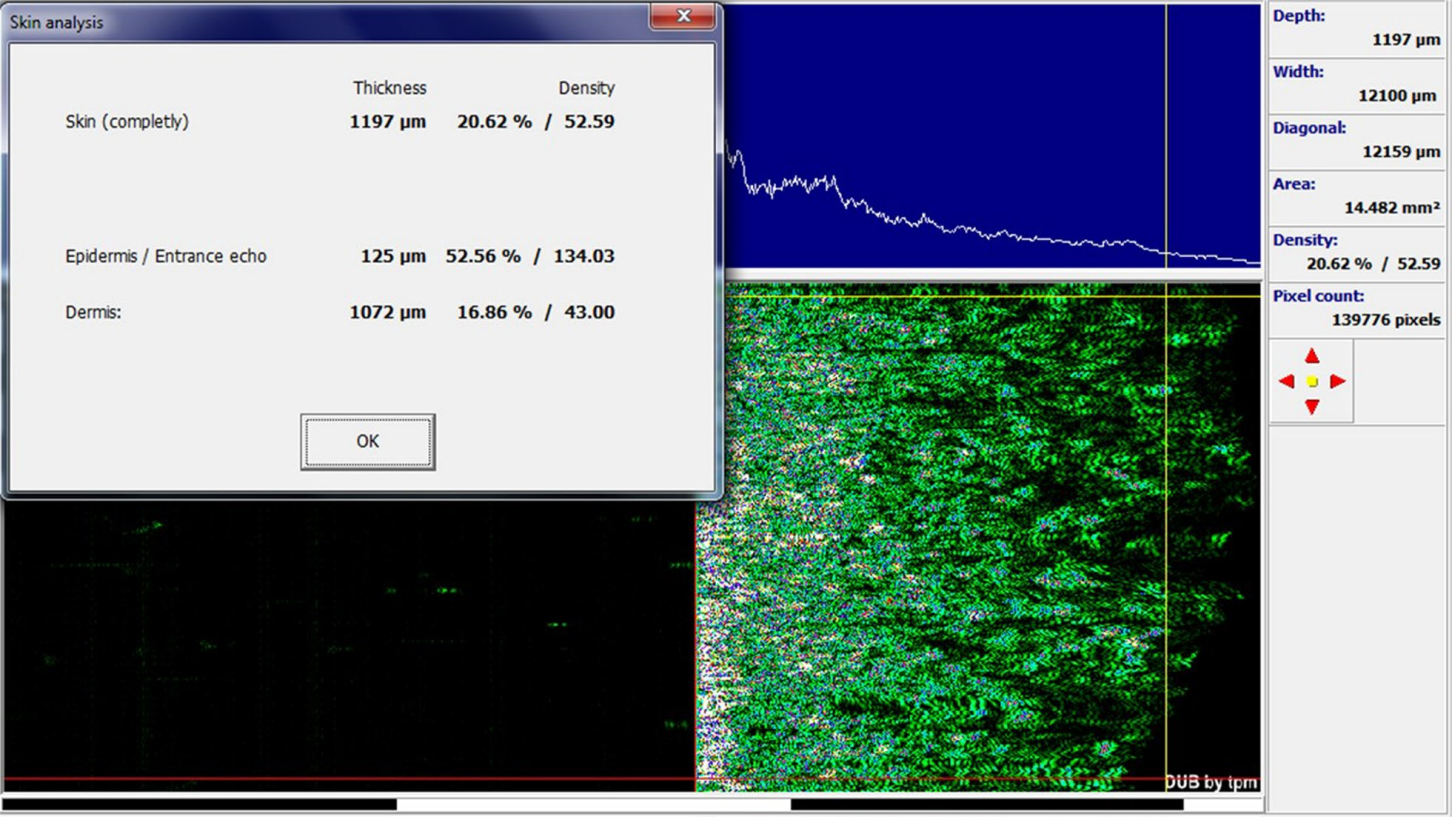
Sonographic findings after intervention with fractional CO_2_ laser and SVF
